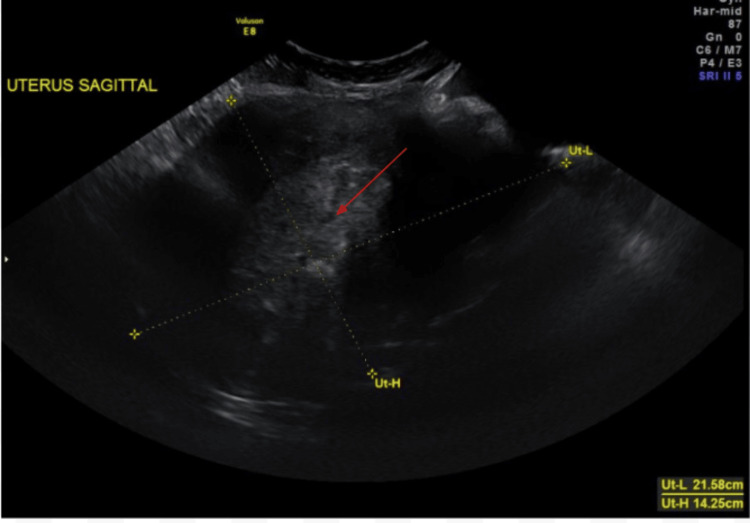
Pelvic ultrasound showing an enlarged fibroid uterus with numerous fibroids and a markedly thickened, heterogeneous endometrium measuring 4.8 cm (thin red arrow)
